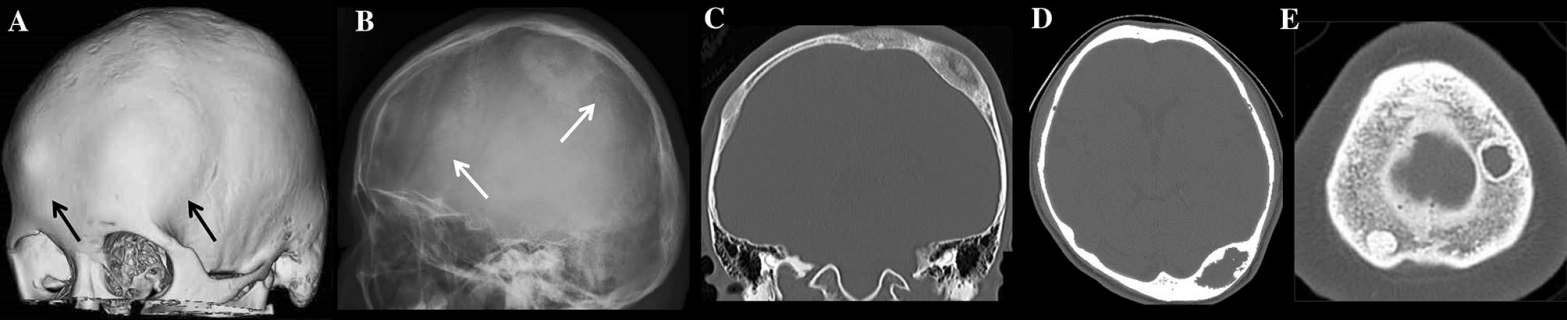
Cranial lesions in two female patients with a novel heterozygous *IFITM5* mutation p.N48S. (A) 3D CT showing osseous bumps of the right frontal and left parietal calvaria (black arrows); (B) skull radiograph showing doughnut lesions of the frontal and parietal regions (arrows), corresponding to ones in (A); and (C) Coronal CT showing parietal hyperostosis with central radiolucency in an 18-year-old female. (D) CT showing calvarial thickening with central radiolucency of the left occipital region in a 47-year-old female. (E) Cross-sectional CT image of an adult male patient with a heterozygous SGMS2 mutation p.R50* showing multiple sclerotic lesions
